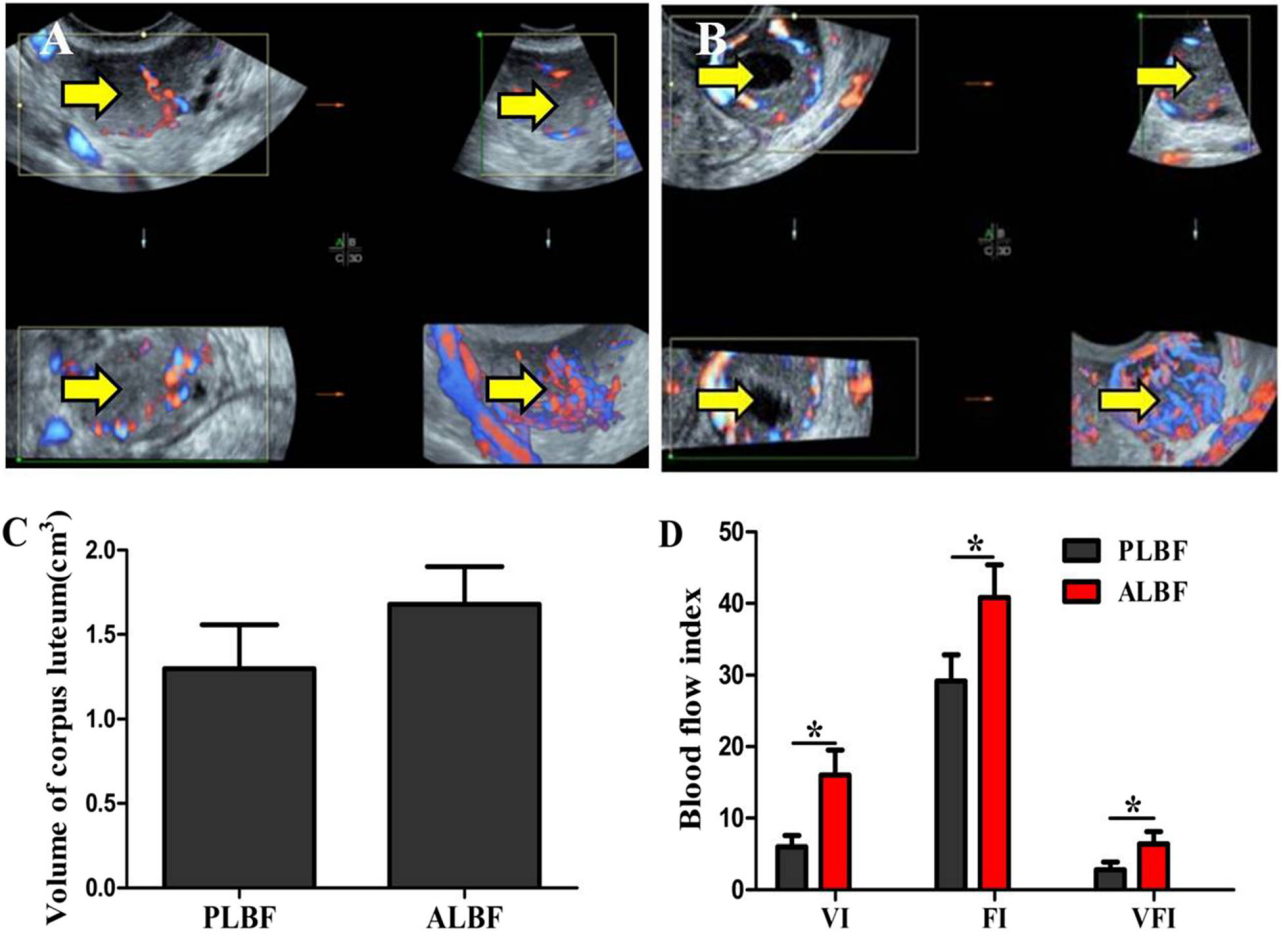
Corpus luteum measured by 3D power Doppler ultrasound. The arrow indicates the corpus luteum (P* < 0.05). PLBF: Poor luteal blood flow group, ALBF: Abundant luteal blood flow group. a Poor luteal blood flow and b abundant luteal blood flow are presented. c and d Comparison of volume, VI, FI and VFI of the corpus luteum measured by 3D power Doppler ultrasound between PLBF and ALBF
